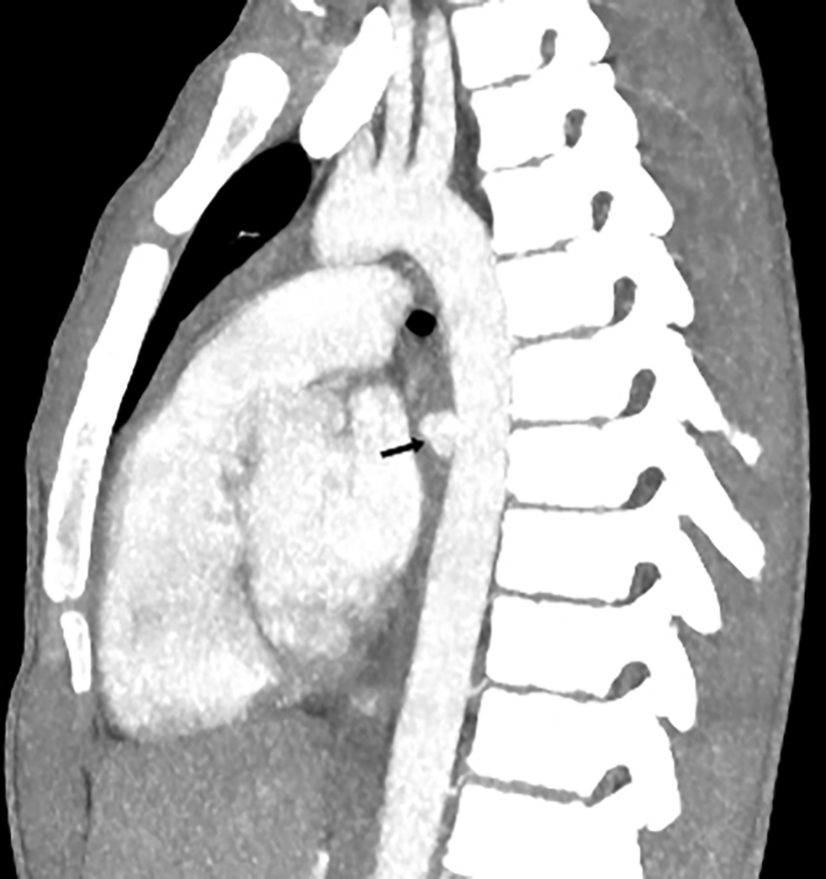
Image of contrast-enhanced computed tomography.
